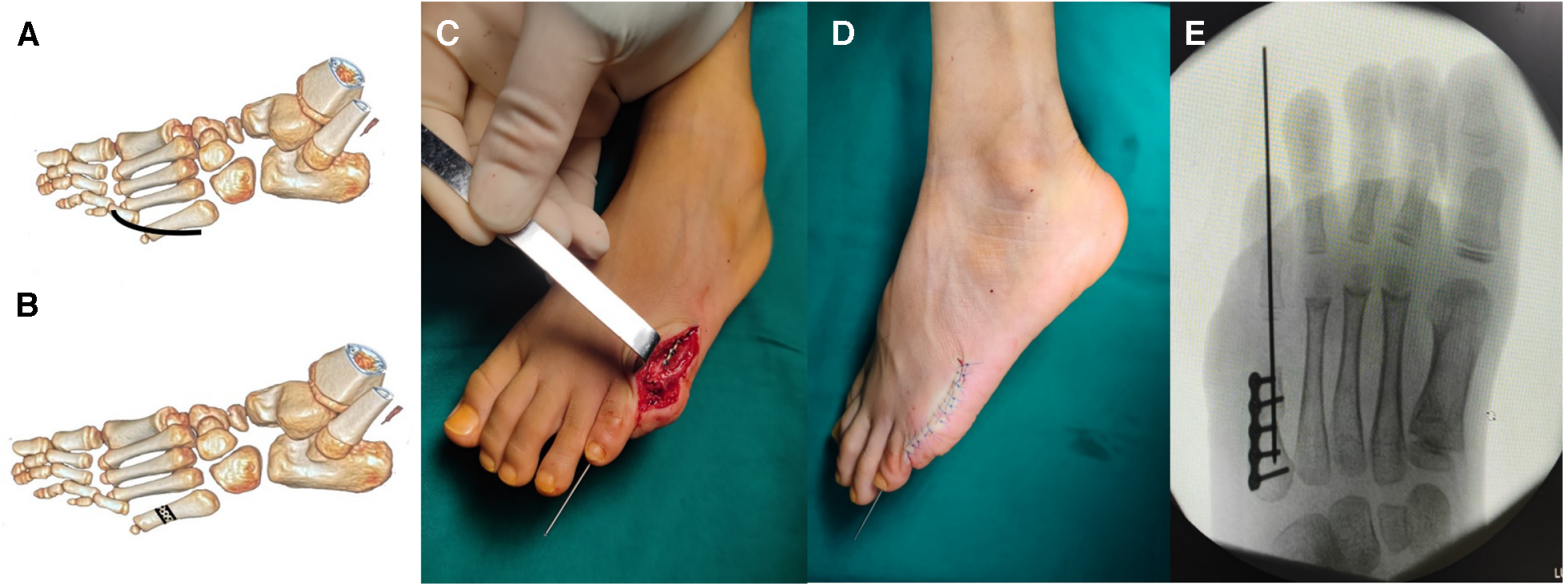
The metatarsal osteotomy procedure. The curvilinear incision was performed over the fifth MTPJ (A); the osteotomy in the metatarsal bone (B); after reduction of the MTPJ, the osteotomy was performed with fixation by the lock compression plate (C); the Kirschner wire was used to keep the stability of the MTPJ (D); the anteroposterior radiograph shows the reduction of the MTPJ (E).
